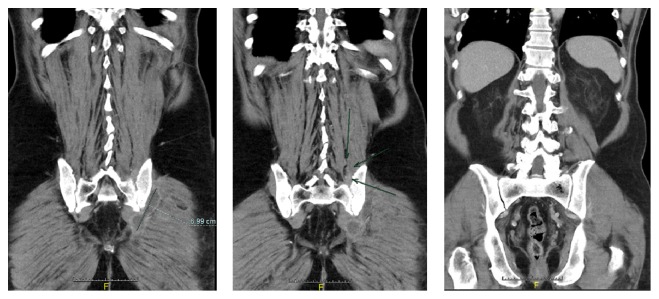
CT scan with multiple free air bubbles in the massa lateralis of the sacrum as well as edema of the iliacus and lumbricales muscles of the left side.
